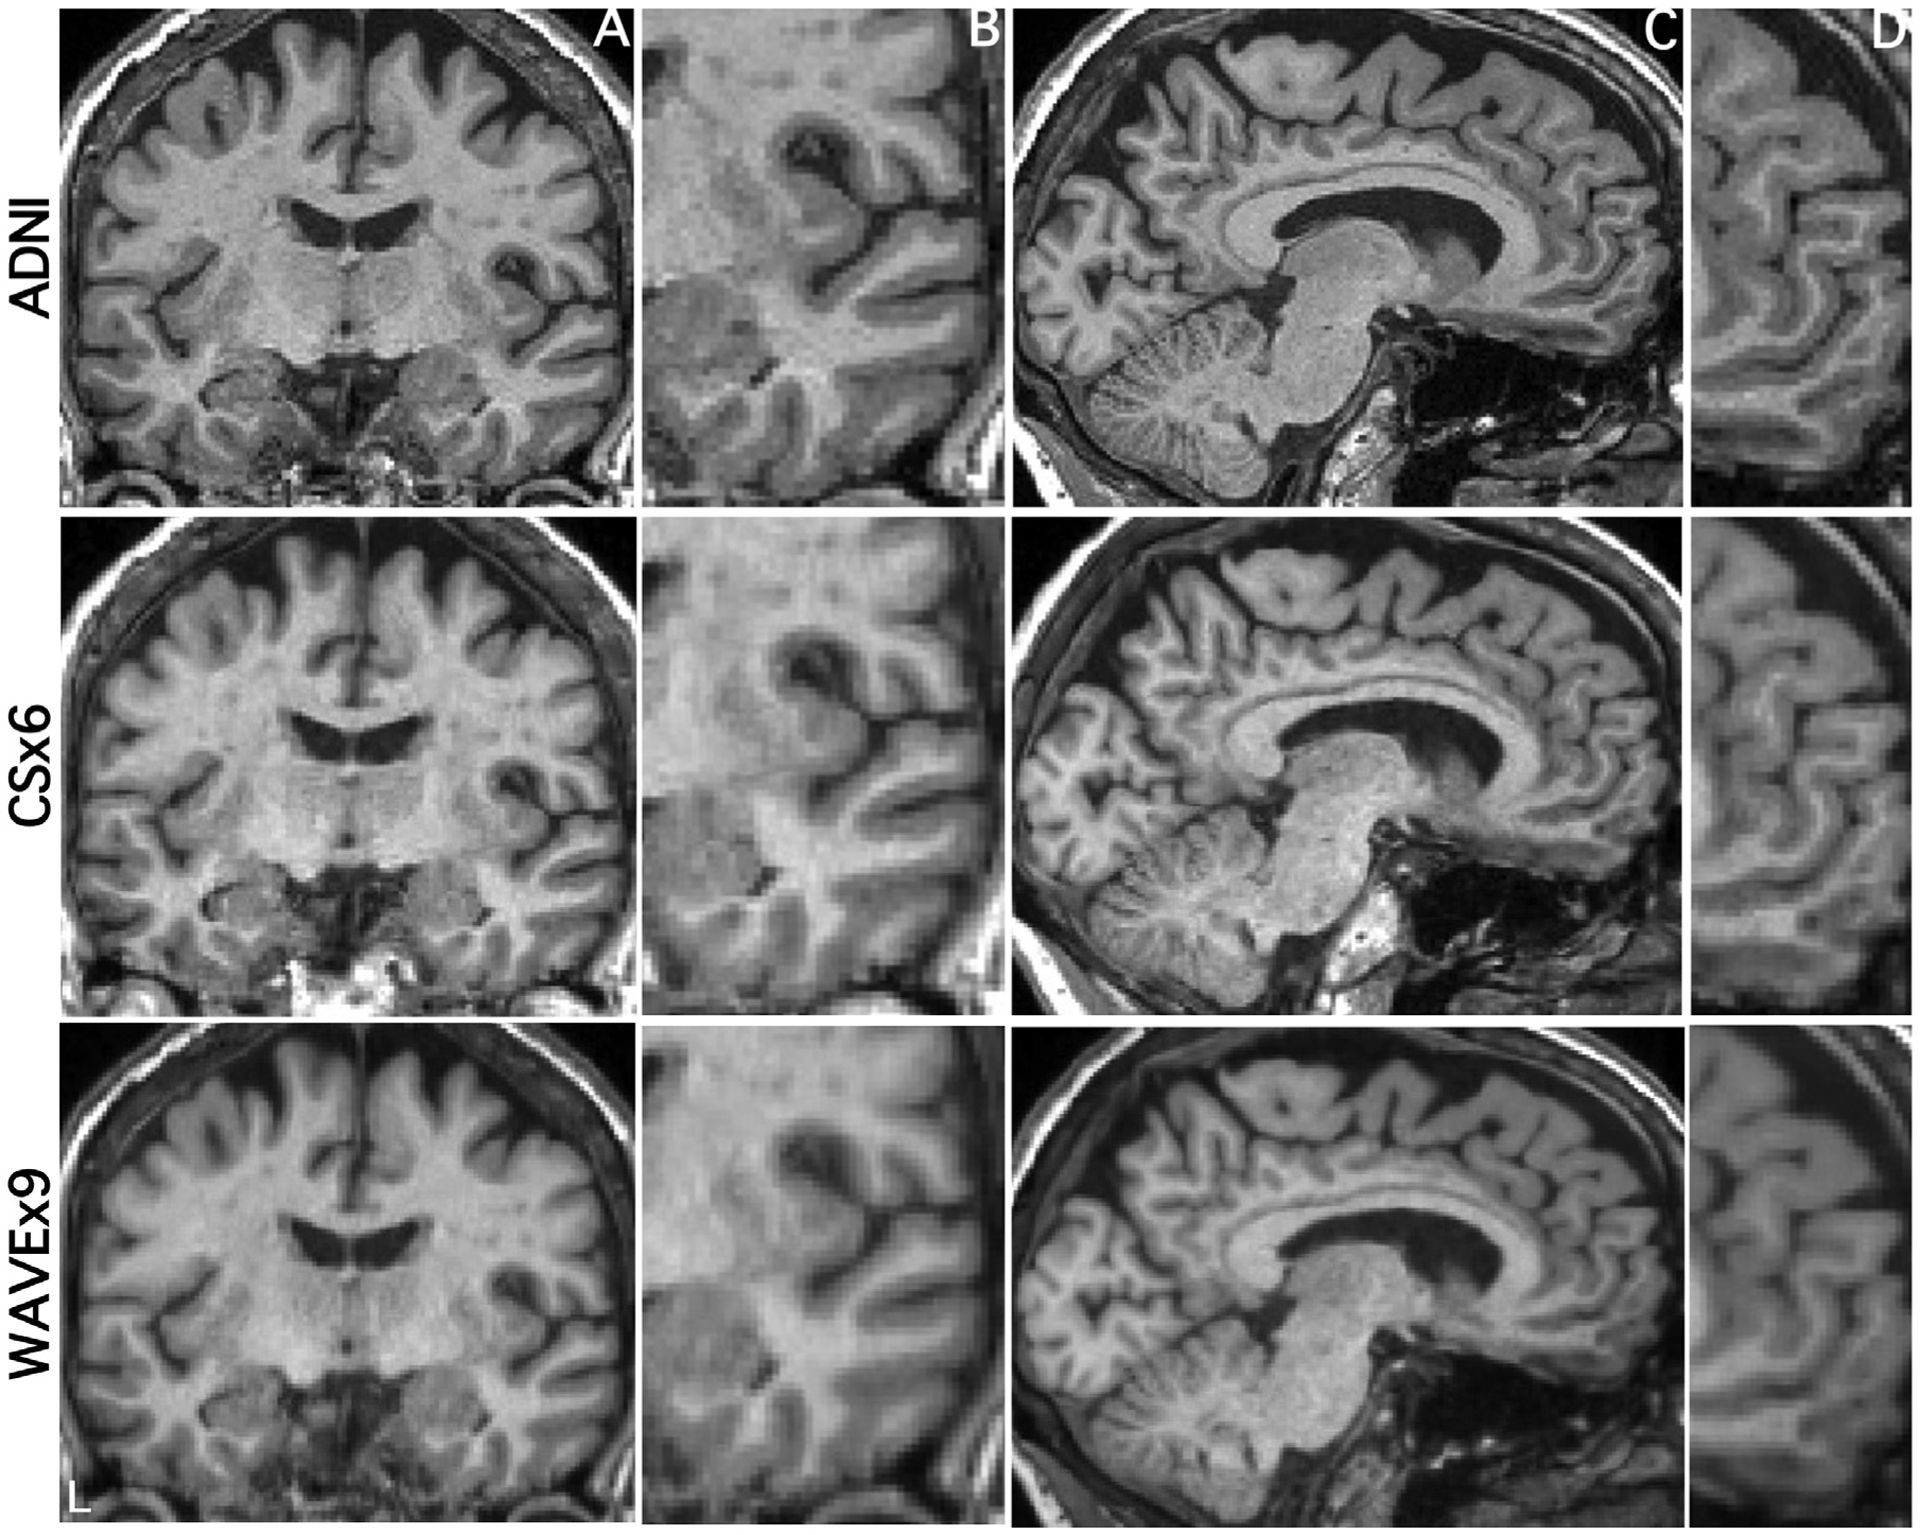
Extremely rapid compressed-sensing and Wave-CAIPI protocols generate morphometrics-ready high-resolution T1-weighted structural scans. The same coronal and sagittal slices (A, C) alongside a zoomed-in portion of each respective slice (B, D) are displayed from the same scan session for three scan types from a single representative participant (77-year-old cognitively unimpaired female). In row 1, images are from a standard 1.0 mm isotropic T1-weighted acquisition (5′12”; ADNI). In row 2, images are from the 1.0 mm isotropic CSx6 acquisition (1′12”). CSx6 achieves a ~5-fold reduction in scan time compared to the standard ADNI acquisition by sparsely sampling k-space data during acquisition. In row 3, images are from the 1.0 mm isotropic WAVEx9 acquisition (1′09”). WAVEx9 achieves a ~5-fold reduction in acquisition time through parallel imaging. Note that while the CSx6 and WAVEx9 achieve high-resolution images, there are differences in image quality when compared with the ADNI image. In B the boundary between the pallidum, putamen, and the surrounding white matter shows lower contrast. In D, the medial prefrontal cortex appears grainier with lower contrast.
